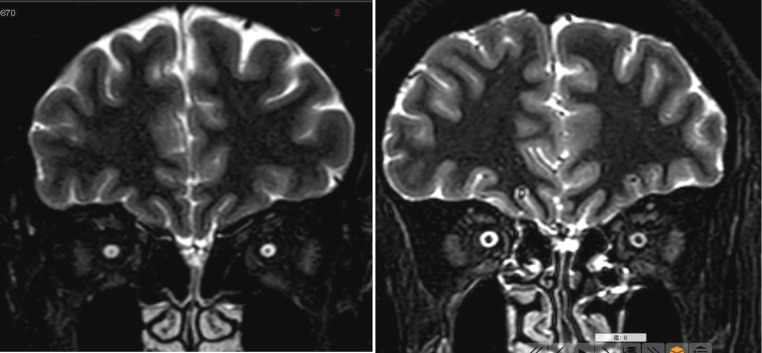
Distension of the perioptic subarachnoid(DPSS) on the coronal T2- weighted orbital MRI. Left: DPSS on both side. Rgiht: DPSS on right side
